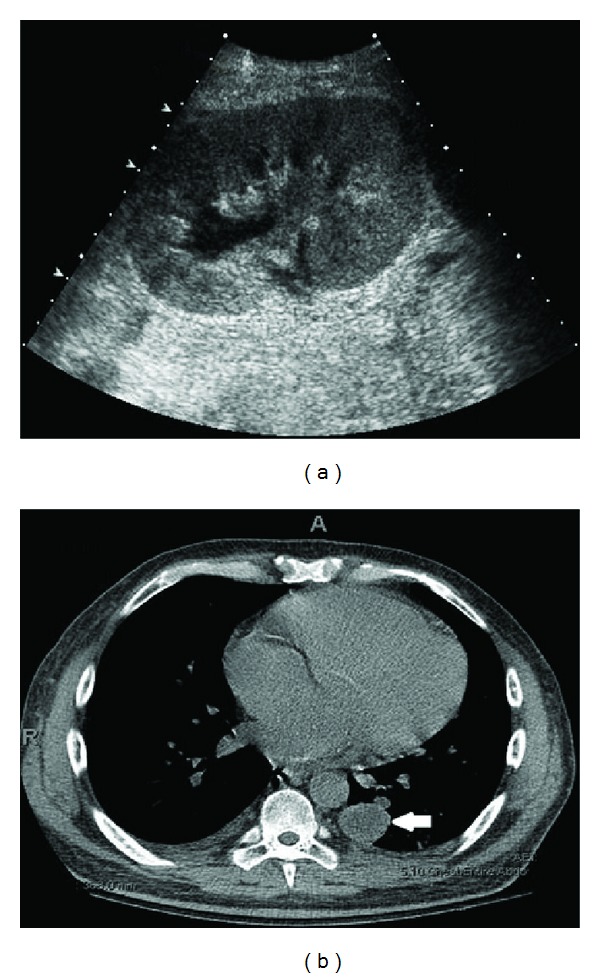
(a) Ultrasound showing enlargement of the transplanted kidney; (b) CT scan of the chest showing a cystic mass in the lower lobe of the left lung (white arrow).
